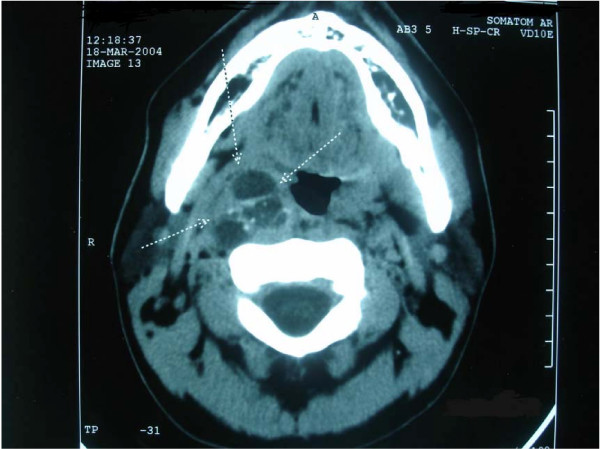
Computed tomography of a patient (GK) with metastasis in the parapharyngeal area from papillary thyroid microcarcinoma (for details see Table 1).
